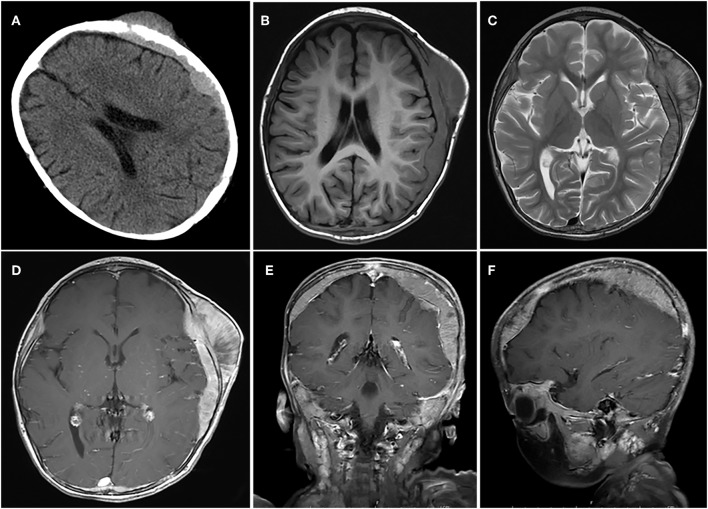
A case of huge tumor. (A) Axial CT scan shows show that the lesion had broken through the outer table of the skull to invade the scalp. (B–F) The tumor was located in the epidural and subdural space with a wide base, adjacent skull erosion, and soft tissue invasion under the scalp.
